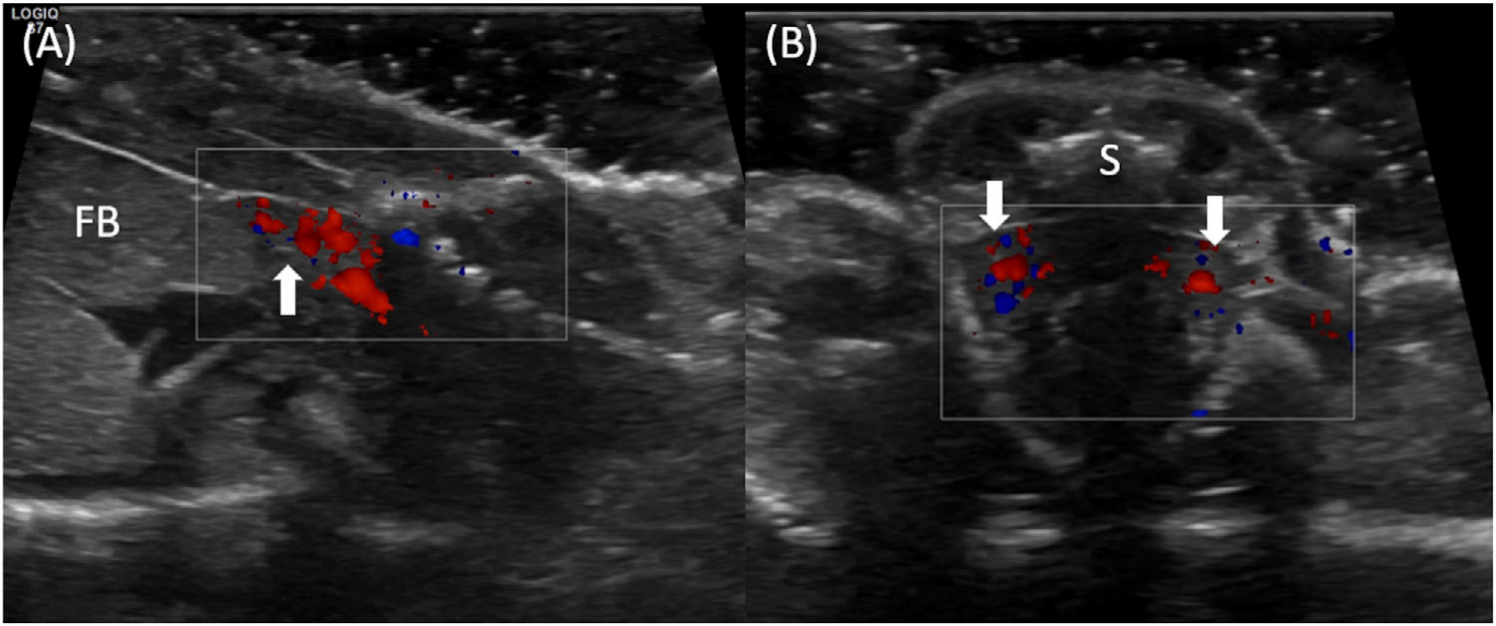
Ultrasound images of the pelvic region of a female leopard gecko using an 18 MHz linear transducer. A, Parasagittal section of the right cranial renal pole (top arrow). Using color Doppler, the renal blood flow can be visualized, and the kidney can be differentiated from the adjacent fat body (FB). B, Transverse section of the left and right kidney (arrows below). Both show good blood flow. The acoustic shadow of the spine (S) can partially obscure parts of the kidneys.
